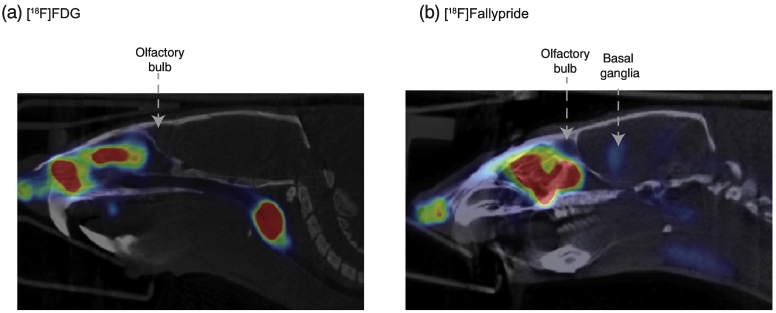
Figure shows representative images of the lateral view of the brain after intranasal administration of (a) [^18^F]FDG and (b) [^18^F]fallypride. The olfactory region is indicated with the arrow in both cases, as well as the basal ganglia in case of [^18^F]fallypride.
